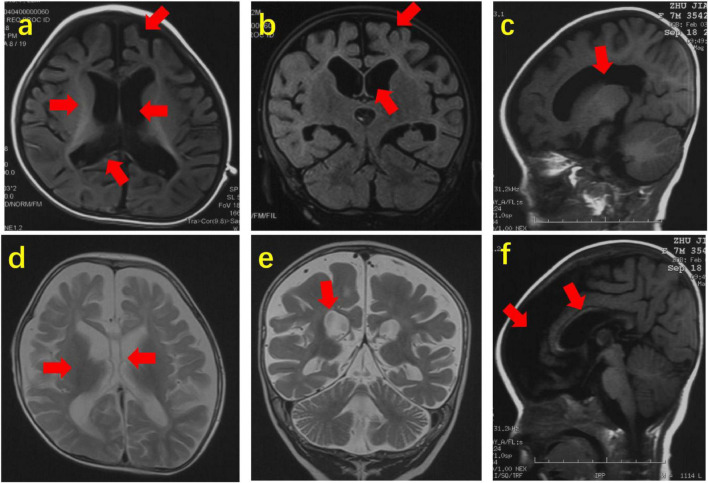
Abnormal brain MRI of 3 patients with *SCN2A* variants. Brain MRI of Patient 14 at the age of 22 months. (a,b). Axial and coronal images (T1WI) showing agenesis of corpus callosum, delayed white matter myelination, dysplasia of frontotemporal lobes and enlargement of lateral ventricles. Brain MRI of Patient 29 at the age of 7 months. (c,f) Sagittal images (T1WI) showing enlargement of lateral ventricles, agenesis of corpus callosum and dysplasia of frontotemporal lobes. Brain MRI of Patient 39 at the age of 12 months. (d,e) Axial and coronal images (T2WI) showing agenesis of corpus callosum, delayed white matter myelination and enlargement of lateral ventricles. The arrow points to the lesion.
